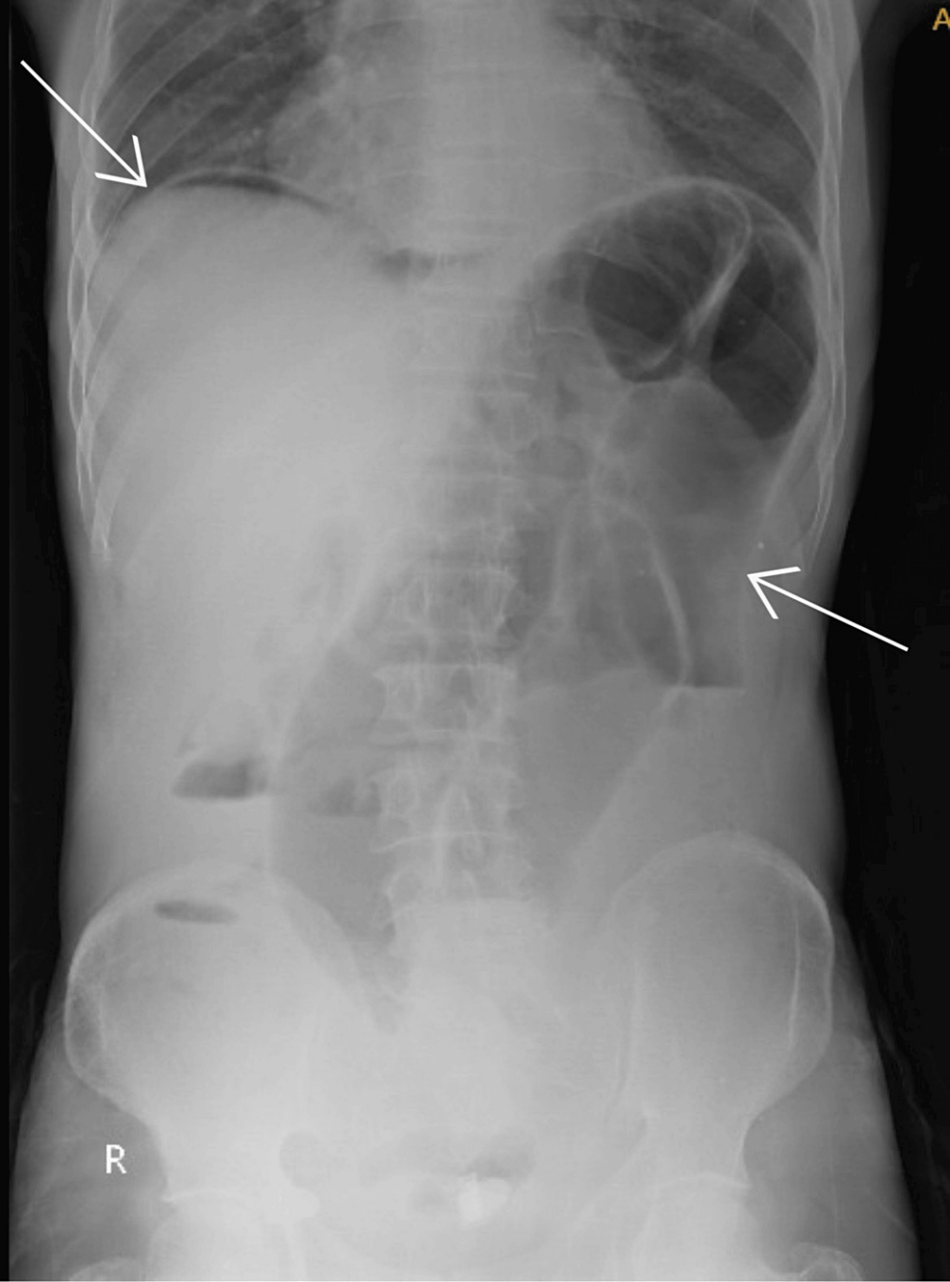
X-ray image suggestive of gas under the diaphragm and sigmoid volvulus
